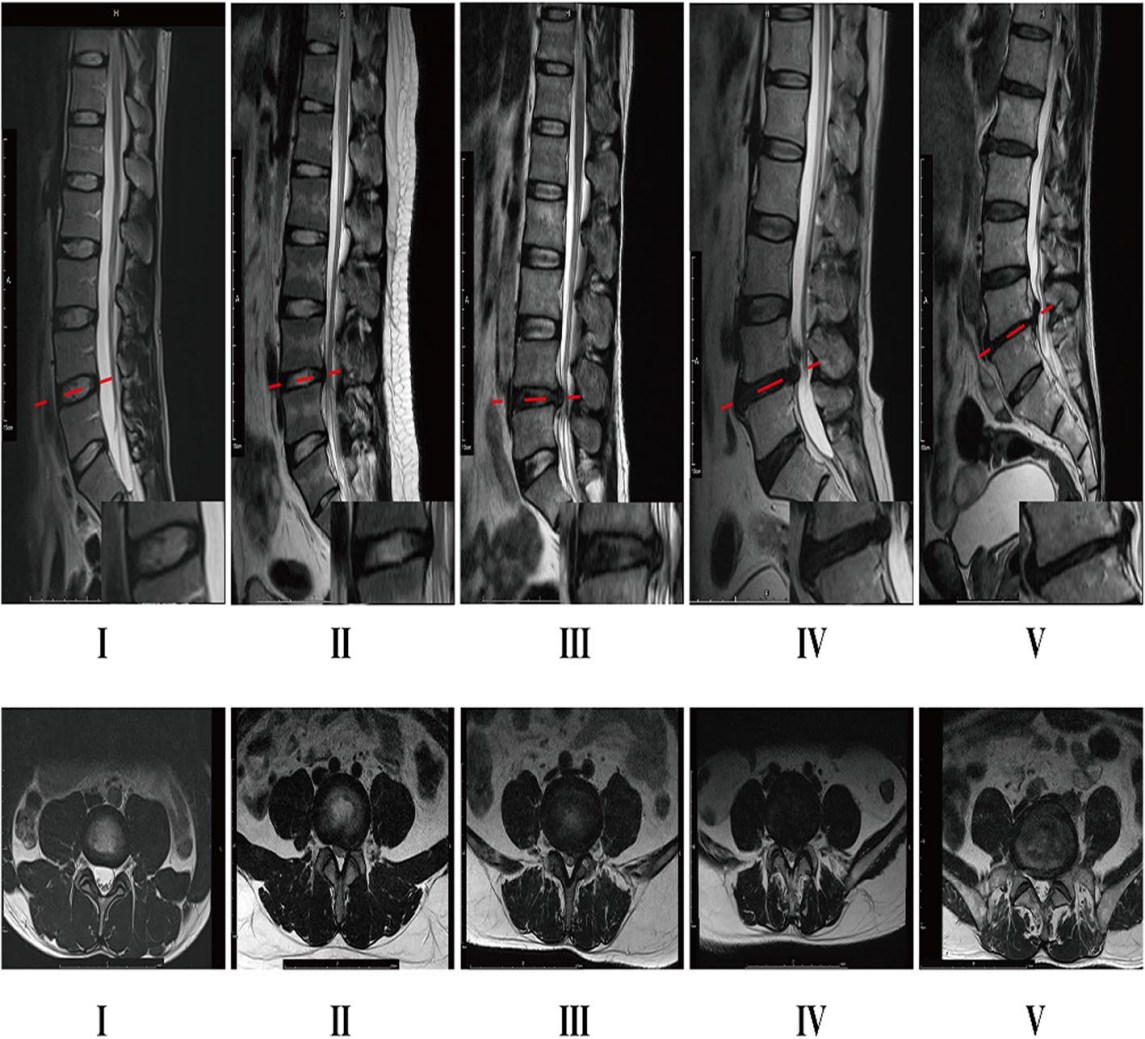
Pfirrmann classification of LDD: Grade I: The structure of the disc is homogeneous, with a bright hyperintense white signal intensity and a normal disc height. Grade II: The structure of the disc is inhomogeneous, with a hyperintense white signal. The distinction between nucleus and anulus is clear, and the disc height is normal, with or without horizontal gray bands. Grade III: The structure of the disc is inhomogeneous, with an intermediate gray signal intensity. The distinction between nucleus and anulus is unclear, and the disc height is normal or slightly decreased. Grade IV: The structure of the disc is inhomogeneous, with a hypointense dark gray signal intensity. The distinction between nucleus and anulus is lost, and the disc height is normal or moderately decreased. Grade V: The structure of the disc is inhomogeneous, with hypointense black signal intensity. The distinction between nucleus and anulus is lost, and the disc space is collapsed. T2-weighted axial images of Pfirrmann Grade of patients
